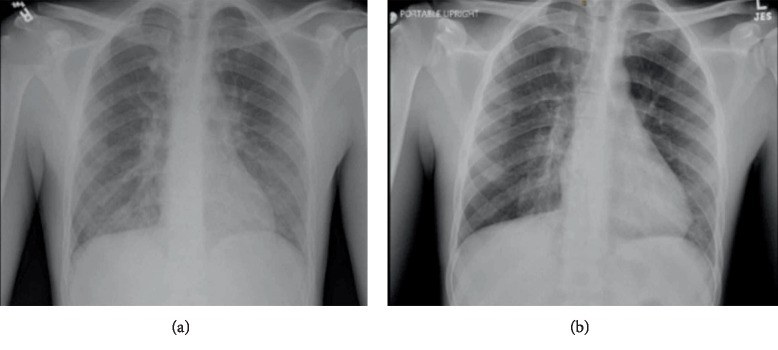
(a) Portable chest X-ray done in the ER on presentation showing bibasilar infiltrates and interstitial prominence. (b) Portable chest X-ray on hospital day 4 prior to discharge with improvement in aeration of the lungs, especially the right lung medially and left lingula. There continues to be minimal interstitial infiltrates present.
